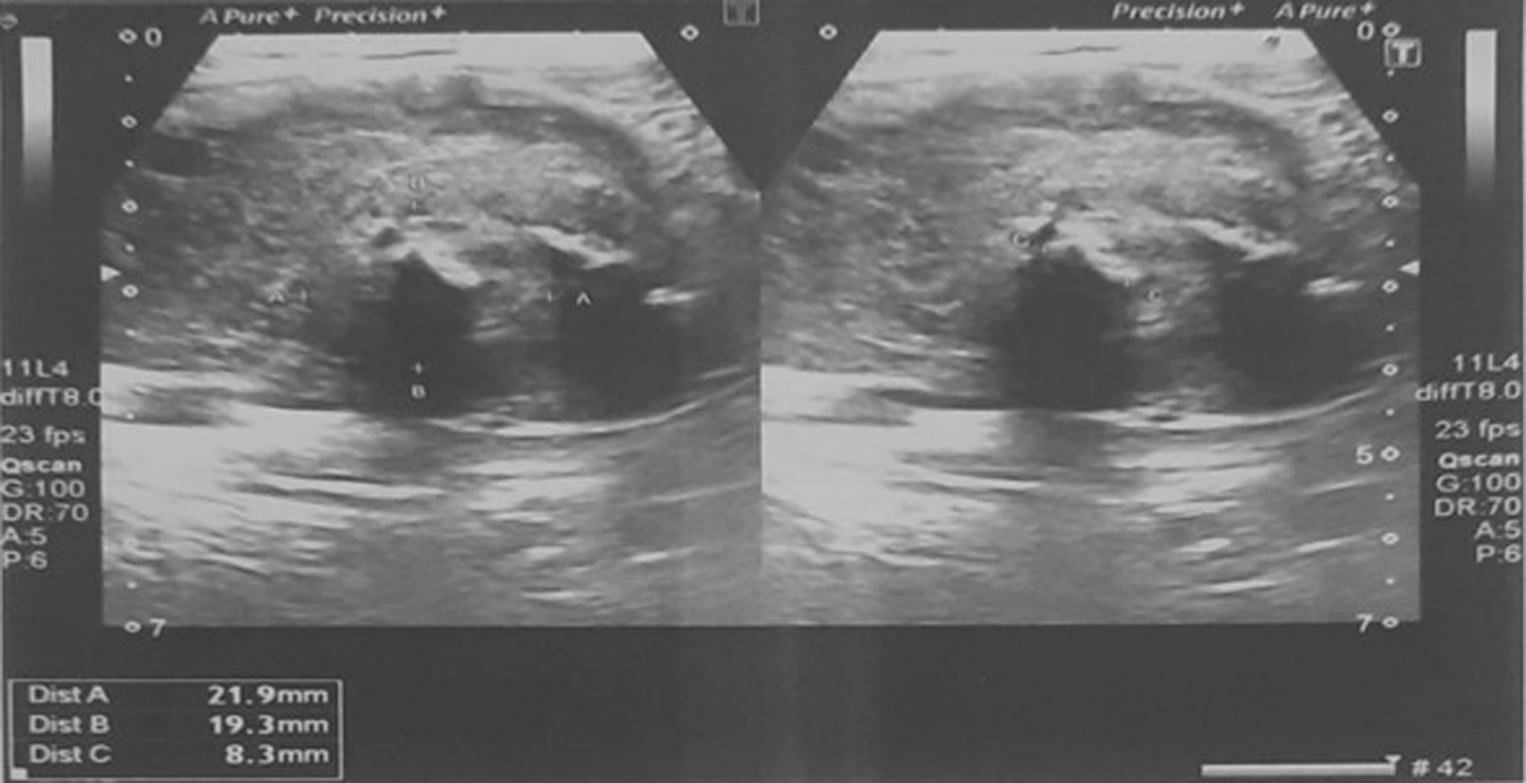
Ultrasound thyroid of a patient with primary leiomyosarcoma of the thyroid.
